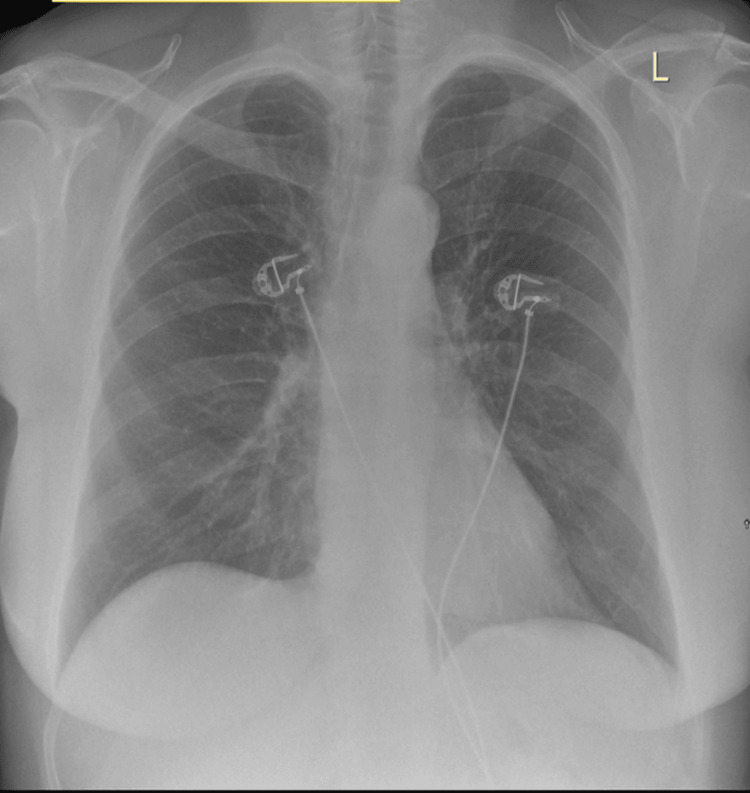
Chest X-ray on presentation showing no signs of consolidation or inflammatory infiltrates.
